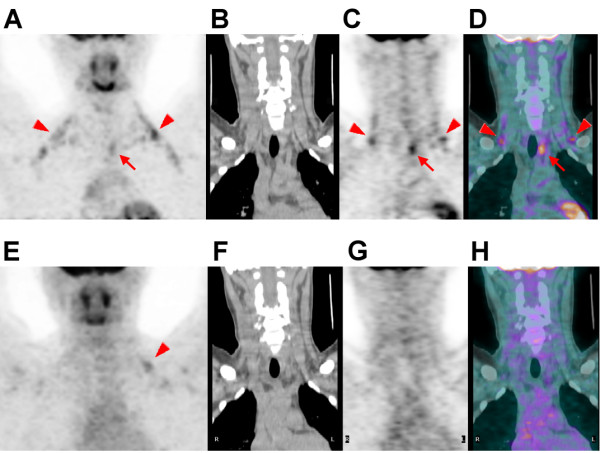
^18^F-FDG PET/CT scan (top row) of an 18-year-old man with a history of papillary thyroid cancer treated with a bilateral total thyroidectomy showing activated BAT deposits in the bilateral posterior neck (PN) and supraclavicular (SC) areas (arrowheads), and a hypermetabolic nodule in the left paratracheal area (arrows). The nodule was surgically excised and proved to be a metastatic lymph node. At that time, the TMA of the BAT was 46.6, the neoplastic status score was 3, the outdoor daily average temperature on the date of the PET/CT study was 25.4 °C, the patient's BMI was 29.0 kg/m^2^, and his fasting blood sugar was 86 mg/dL. On the follow-up ^18^F-FDG PET/CT scan (bottom row) after the lymph node had been surgically excised, there was no abnormal tumor uptake and only minimal activated BAT deposits in the SC areas (E, arrowhead). At that time, the TMA of the BAT was 9.2, the neoplastic status score was 1, the outdoor daily average temperature on the date of the PET/CT study was 24.4 °C, the patient's BMI was 28.1 kg/m^2^, and his fasting blood sugar was 99 mg/dL. (A, E: maximum intensity projections; B, F: coronal CT images; C, G: coronal PET images; D, H: coronal PET/CT fusion images).
